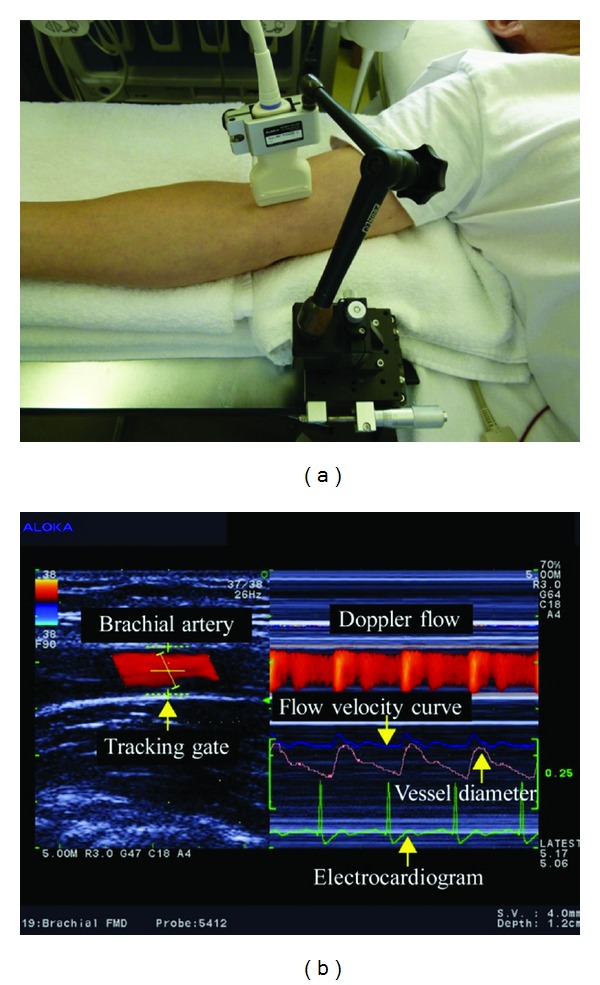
(a) Ultrasound measurement of the brachial artery. 13 MHz linear transducer is fixed along brachial artery with a special probe holder (MP-PH0001, Aloka Co., Ltd., Tokyo, Japan). (b) Display of CDI. Left: image of the vessel image and position of the artery tracking gate. Right: changes in vessel diameter, Doppler flow, and flow velocity, as determined by an automated edge detection device and computer analysis software (e-Tracking system; Aloka Co., Ltd., Tokyo, Japan).
